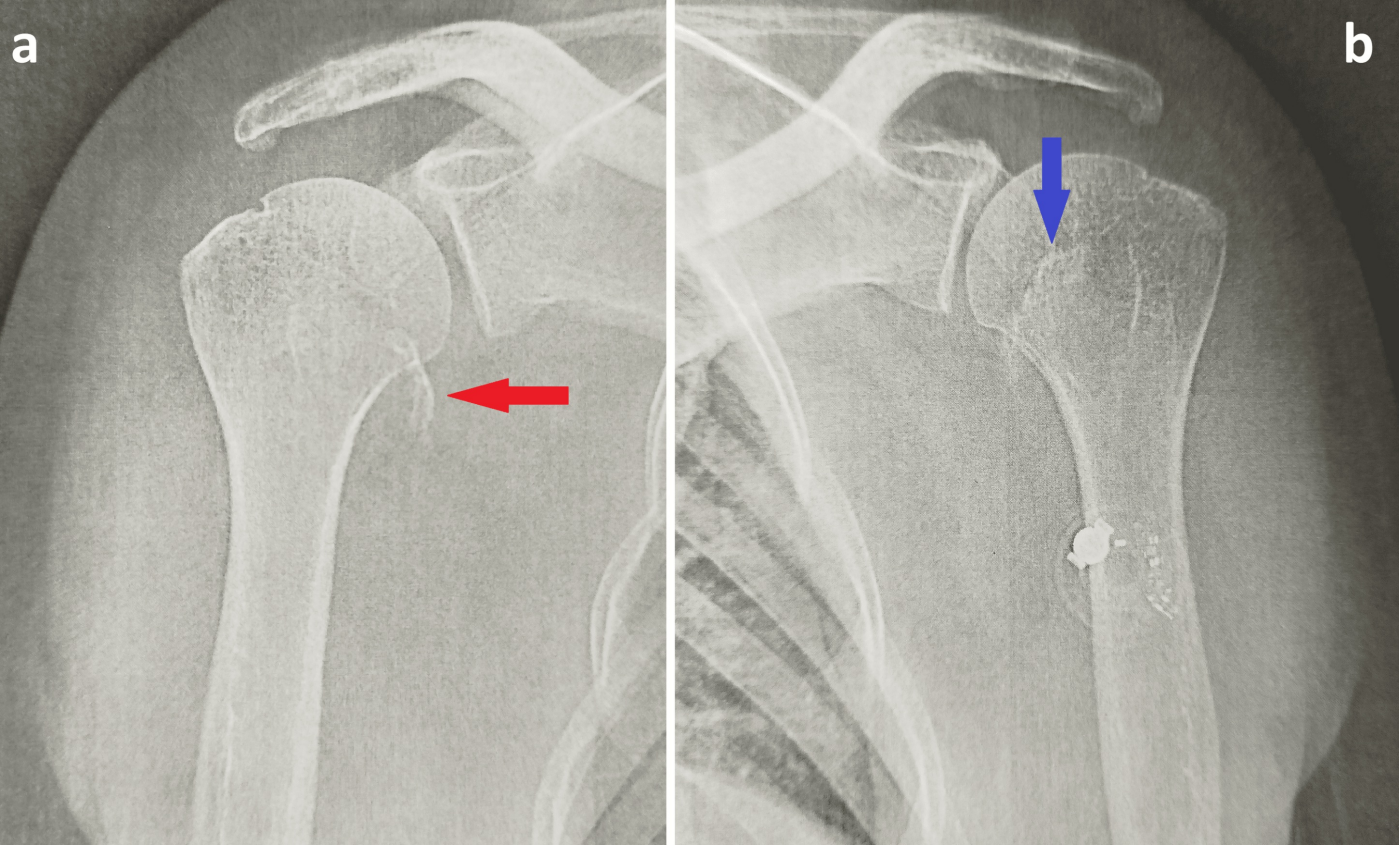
Anteroposterior radiographs of the right (a) and left (b) shoulders demonstrating displaced lesser tuberosity avulsion fractures (red and blue arrows), which are particularly subtle on the left side (blue arrow).
